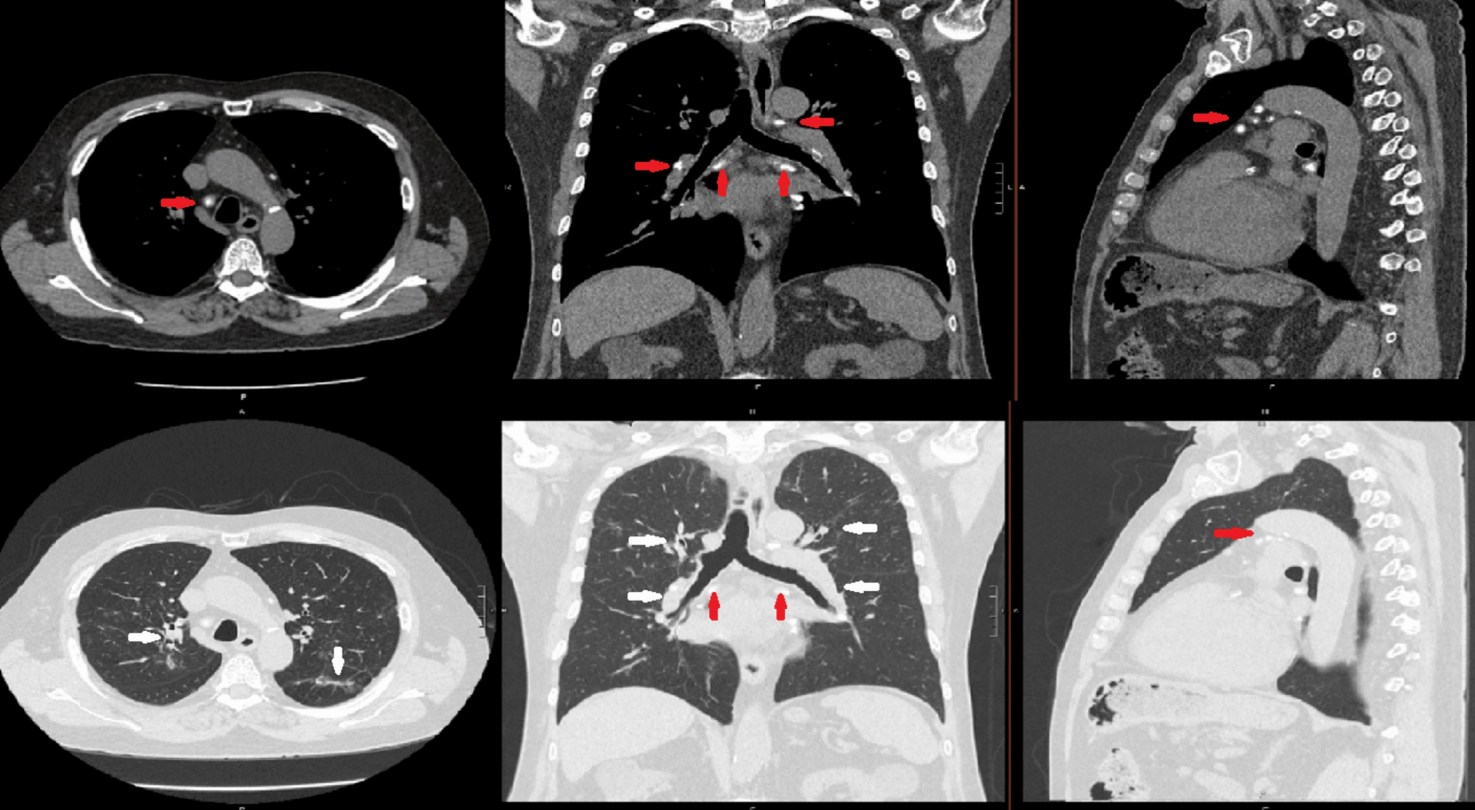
Axial, sagittal, and coronal chest computed tomography images, in mediastinal and lung windows, demonstrating stable calcified mediastinal and hilar lymphadenopathy, marked by red arrows, and persistent, mostly perihilar and perilymphatic nodular disease, marked by white arrows.
